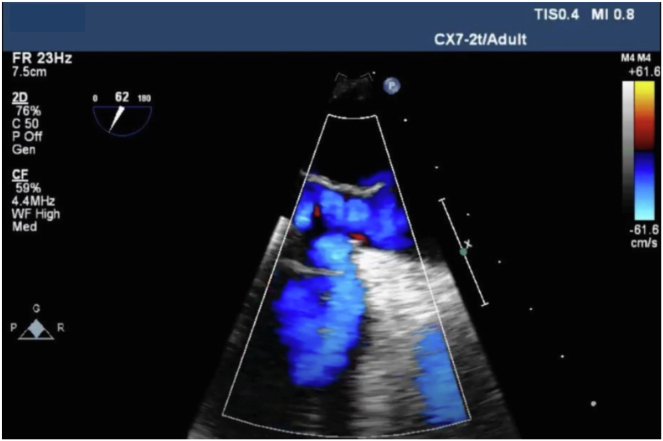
Patent foramen ovale detected during intraoperative transesophageal echocardiogram.
